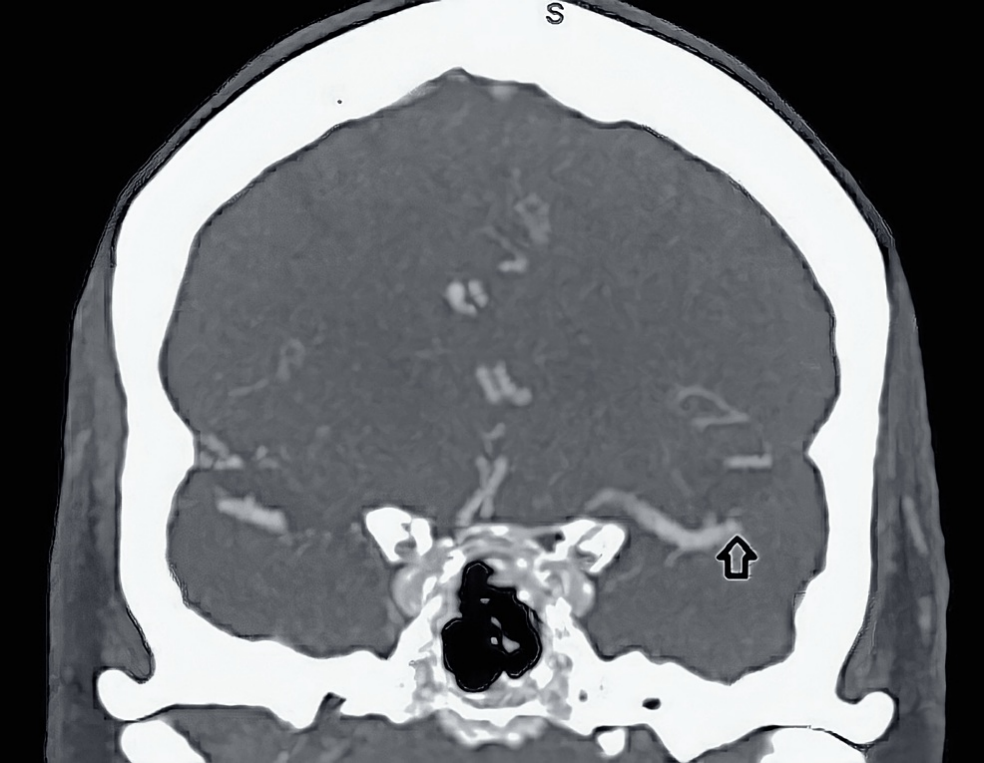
Patient's computed tomography angiogram (CTA) CTA imaging demonstrated stenosis of the M2 segment of the left middle cerebral artery.
